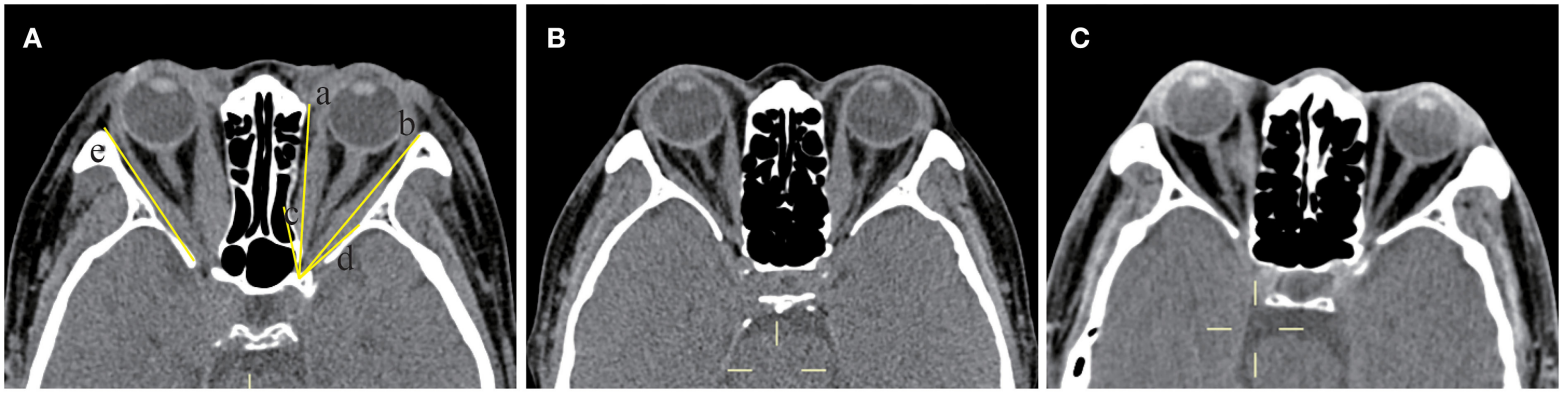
Computed tomography (CT) images at the axial section of bony orbital angles measurement. (A) The orbit with DON, which labels the measurement of the bony orbital angles. The orbital rim angle (ORA) was measured at the level of the medial canthus ligament, measuring the angle (degrees) between two lines, with line a drawn along the margin of the medial orbital wall and line b connecting the frontozygomatic process and the most medial portion of the inner border of the sphenoid wing with extension intracranially until it intercepts with line a. The same axial section containing the bulk of the medial and lateral rectus muscles showed the medial wall angle (MWA) between lines a and c, the lateral wall angle (LWA) between lines b and d, and the orbital apex angle (OAA) between lines c and d. The length of the lateral orbital wall (LWL) was measured on the section just inferior to the anterior clinoid (labeled line e). (B) The orbit without DON. (C) The orbit of the normal control (right eye).
